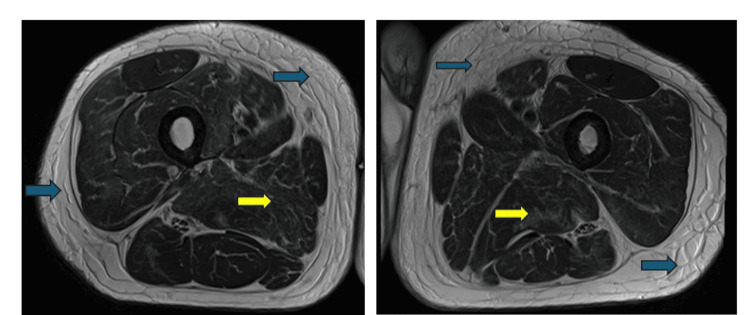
Transverse MRI section through both thighs revealed subcutaneous tissue edema (blue arrows) and edematous changes affecting multiple muscle compartments (yellow arrows).
